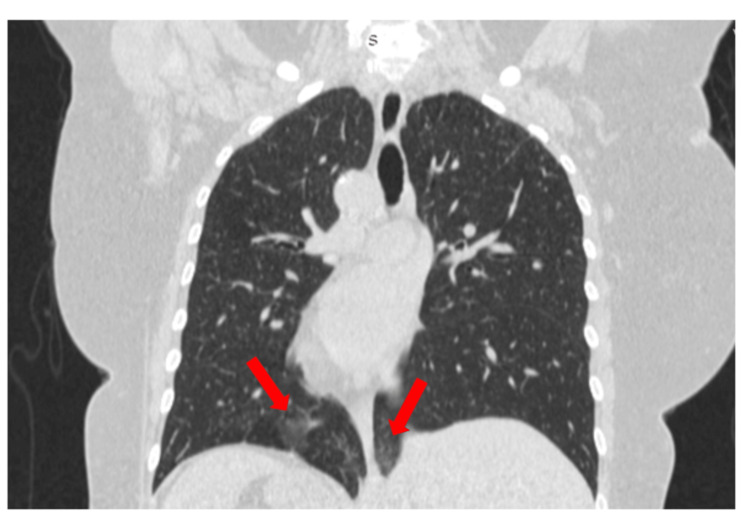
Computed tomography of the thorax, coronal view. Bilateral ground glass infiltrates (arrows) in the lower pulmonary lobes.
